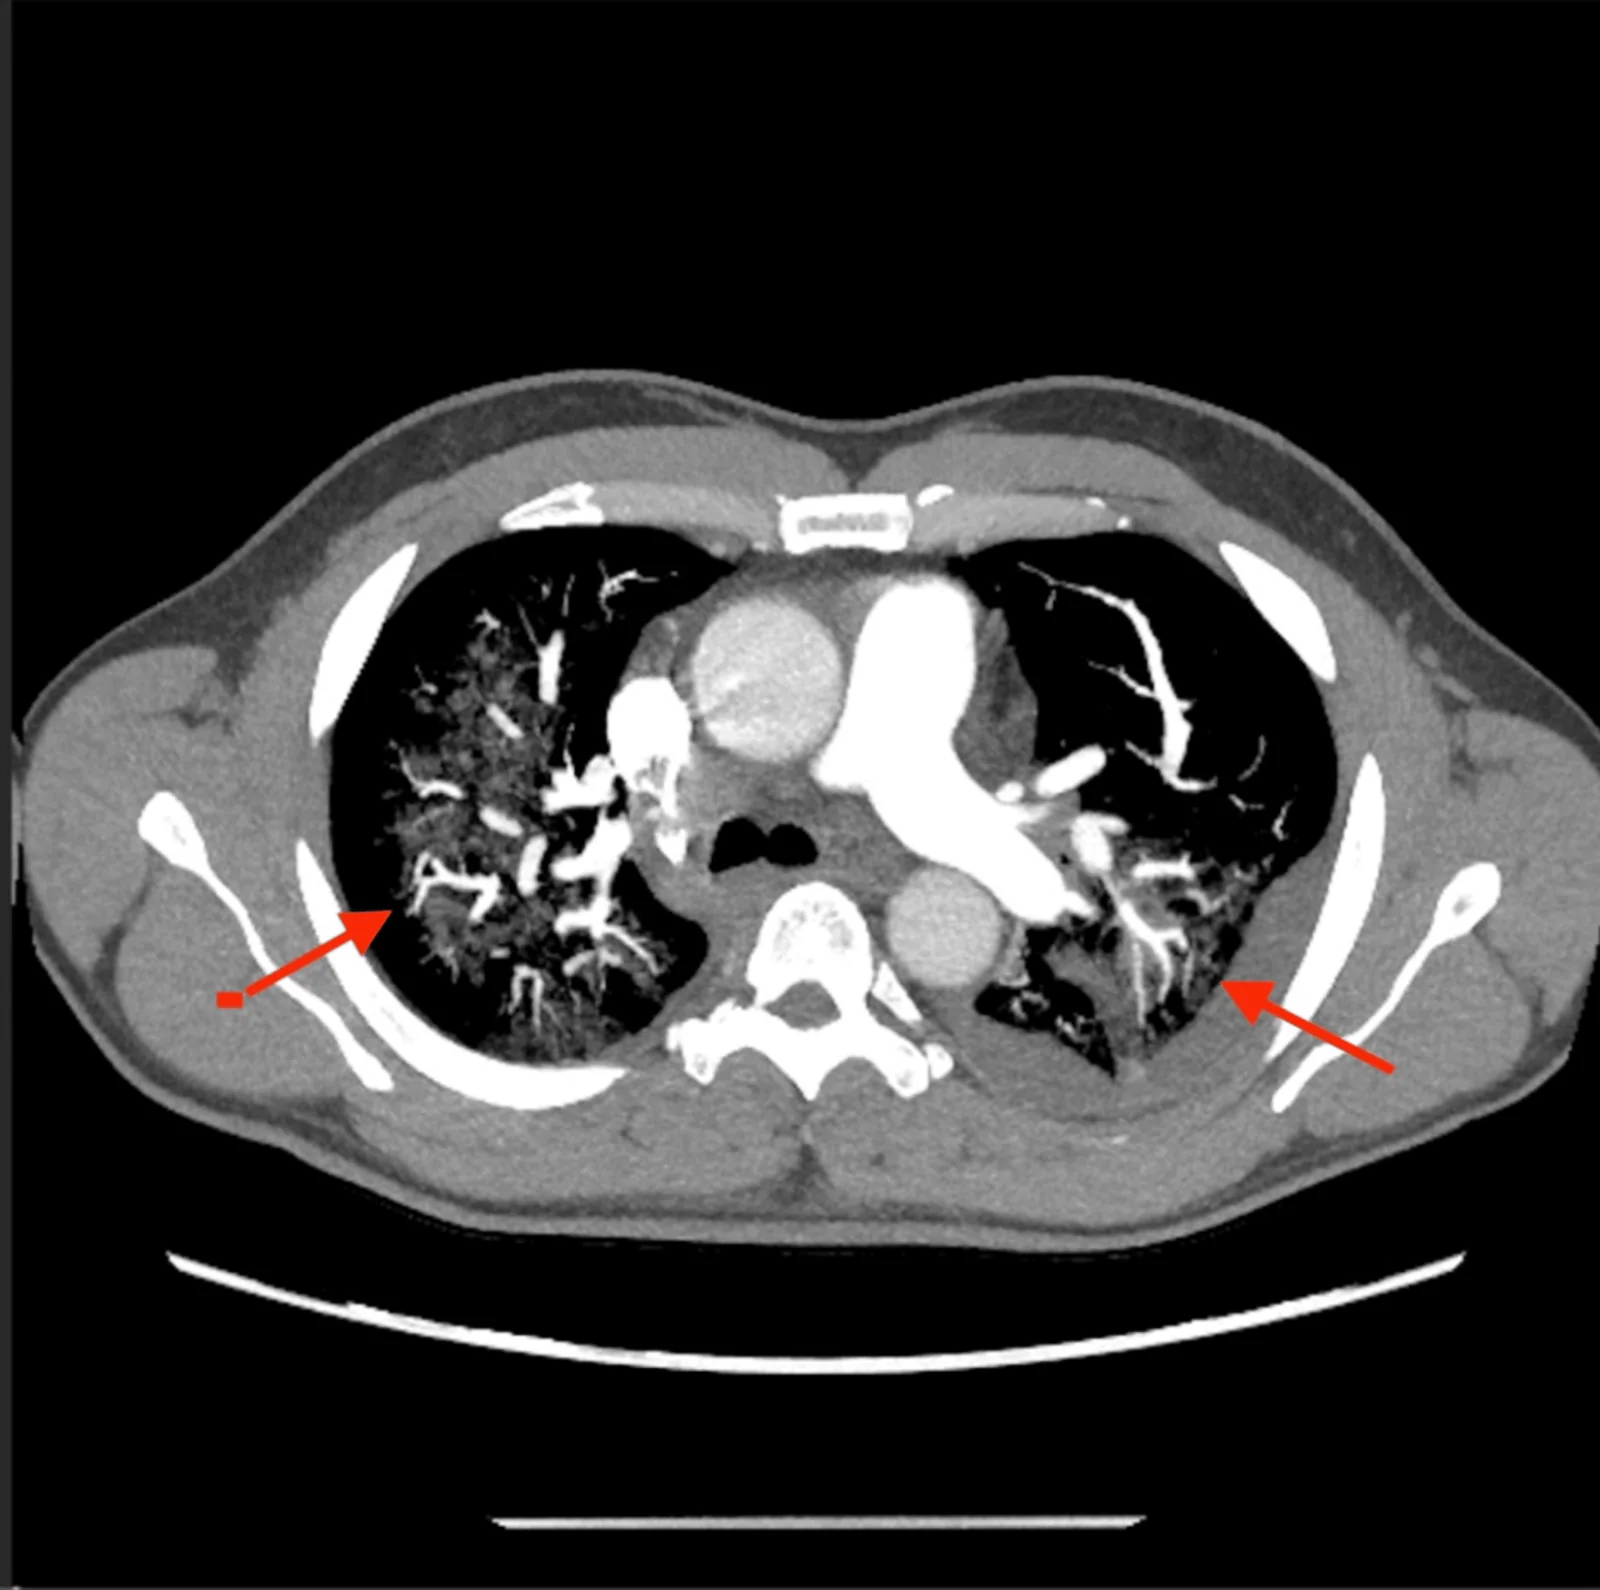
CT chest pulmonary angiogram Bilateral perihilar ground-glass opacities and interlobular septal thickening are observed (red arrows).
